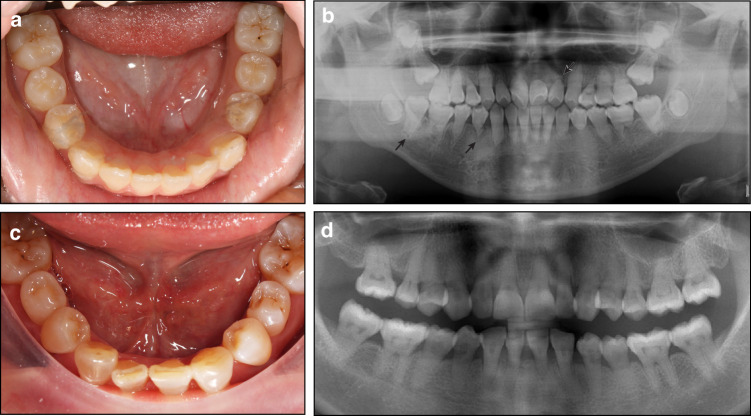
Dentin dysplasia (DD). a, b DD-I in a 13-year-old female. a No obvious abnormalities were observed in the appearance of the teeth. b The radiograph shows short, tapered tooth roots, with the pulp chambers obliterated in all teeth except for the crescent-shaped pulp chamber of tooth 24. No root canal images were observed. Black arrows showed the representative periapical radiolucencies of the non-carious teeth. c, d DD-II in a 33-year-old female. c The teeth exhibit slight yellow discoloration. d The radiograph reveals obliterated root canals and thistle tube-shaped pulp chambers with pulp stones
